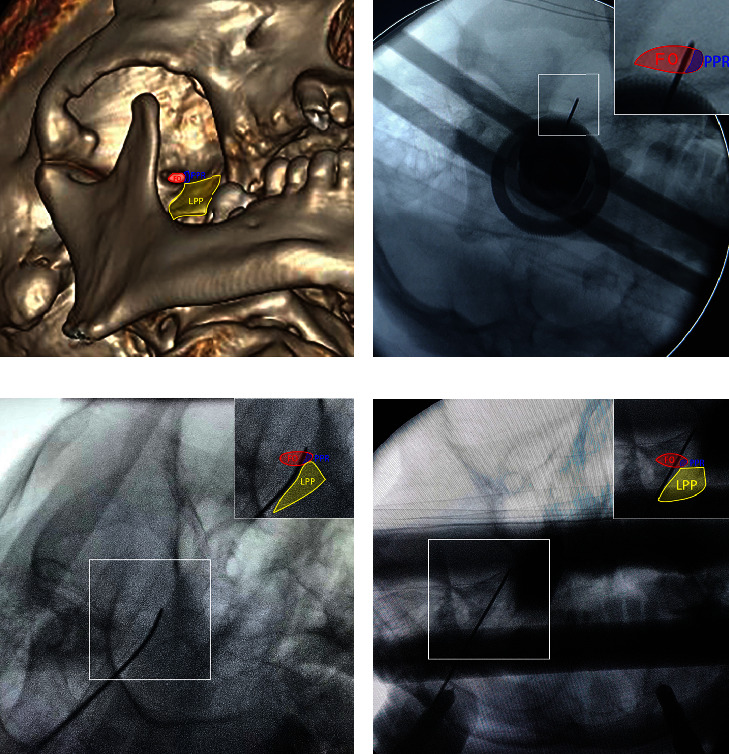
Probe placement into the FO confirmed by C-arm radiography. (a) Three-dimensional computed tomography reconstruction images show the anatomical adjacency among the FO (red shadow), PPR (blue shadow), and LPP (yellow shadow). (b) The first puncture into the FO shows that the probe reached the FO successfully and that the probe was straight. (c) The second puncture to the FO. We decreased the arc angle, and the probe was curved due to being obscured by the PPR. (d) The third puncture to the FO. We increased the arc angle to reach the TGT with the probe through the FO.
